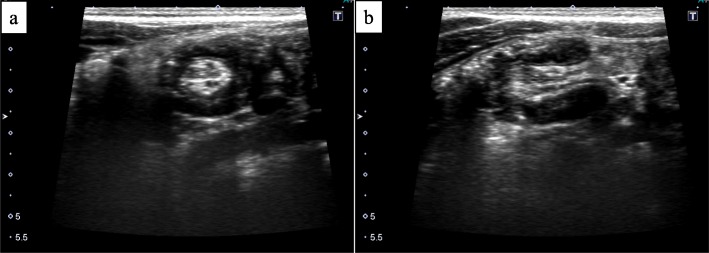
Ultrasonography of the abdomen. a Axial view of the base of the appendix showed target sign-like appearance. b Longitudinal view showed pseudo-kidney sign
